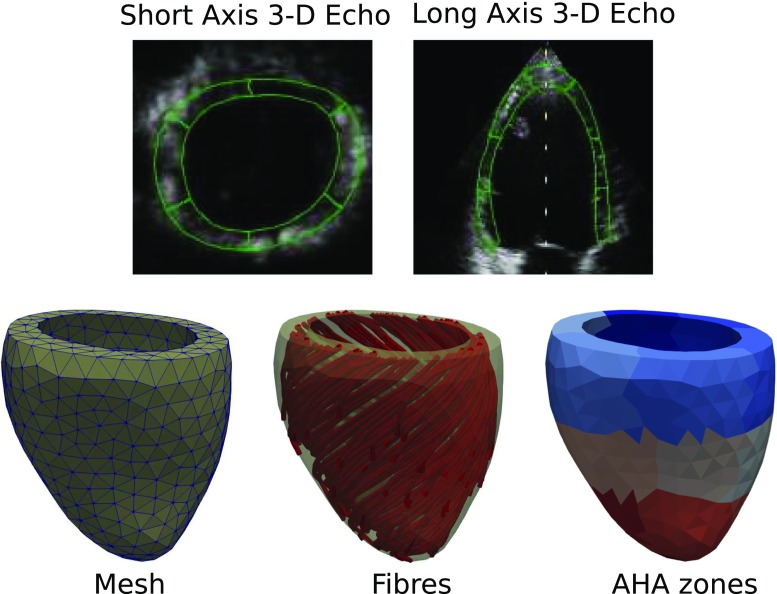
Top row: Example short- and long-axis slices taken from 3-D echocardiography with tracked segments in green. Bottom row: Model LV geometry derived from the 3-D echo data. From left to right are the computational mesh, rule-based fibre orientations and the standard AHA zones shown in separate colours
